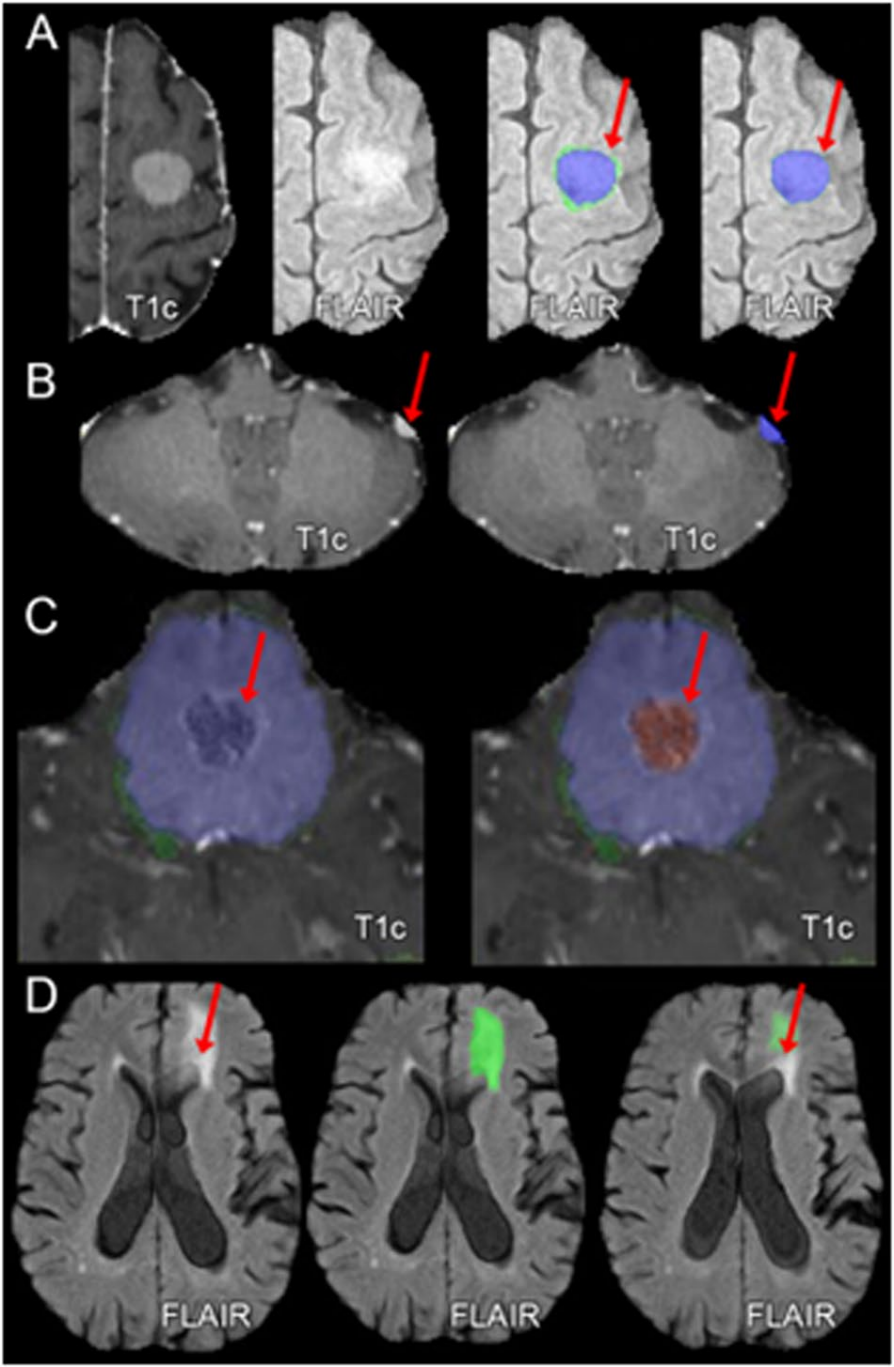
Examples of common errors of automated meningioma segmentation. (A) Erroneously marked a thin rim of edema that does not exist; (B) Missed small convexity meningioma; (C) Improper classification of non-enhancing tumor; (D) Tumor-related edema adjacent to presumed microvascular ischemic periventricular white matter FLAIR abnormalities. T1c: T1-weighted post-contrast imaging; FLAIR: T2-weighted fluid attenuated inversion recovery imaging.
